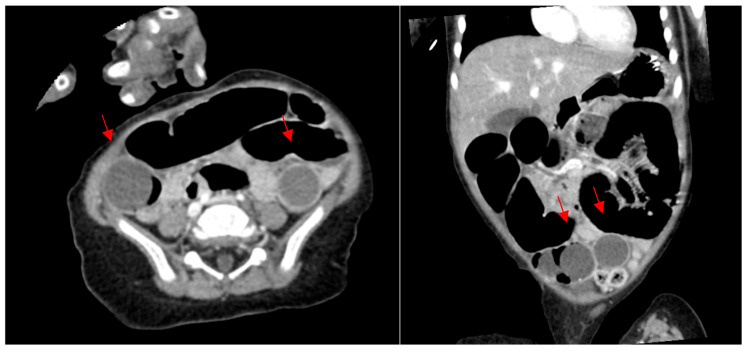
Preoperative computed tomography scan on a 14-month-old patient after water bead ingestion. Arrows indicate expanded beads.
